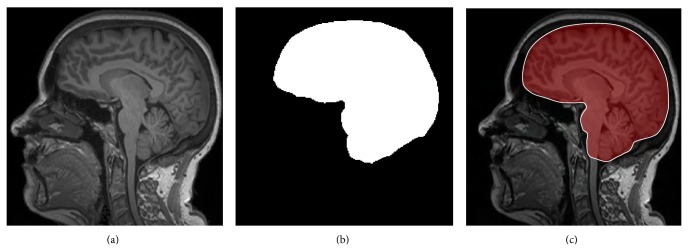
Result of brain extraction on a T_1_ MR image in an axial plane. (a) shows the original T_1_-W MRI. (b) depicts the estimated brain mask. (c) presents an overlap of the brain mask and original MR image.
